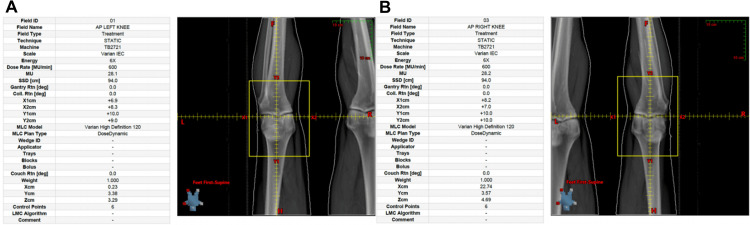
Field verification Digital radiograph of field setup for (A) left and (B) right knee portals.
